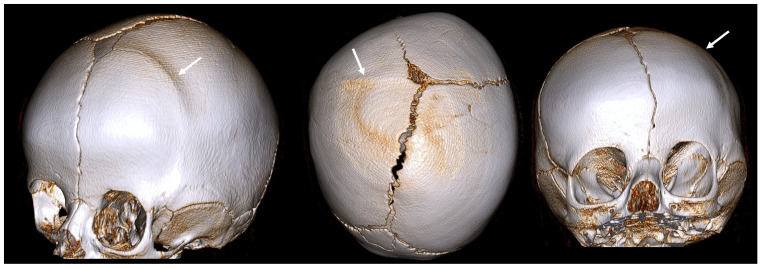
A 3D CT image of a unilateral coronal CRS (anterior plagiocephaly) with an asymmetrical forehead, a flattened forehead and brow on the affected side, an elevated eye cavity on the affected side, and a nose that deviated toward the affected side. The white arrow indicates an abnormally fused suture.
